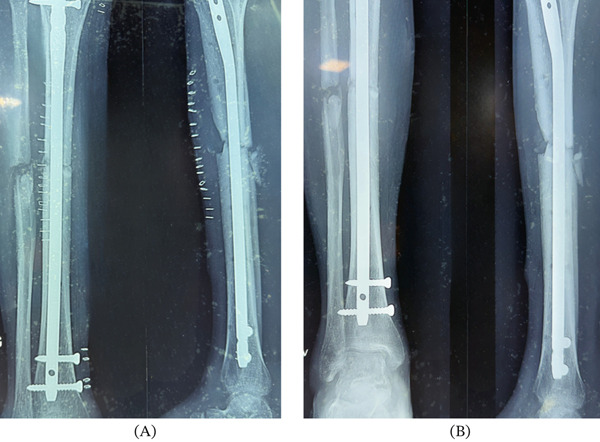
Definitive fixation with intramedullary nailing. (A) Anteroposterior and lateral radiographs dated February 17, 2025, showing intramedullary interlocking nailing with satisfactory alignment and stable fixation. (B) Anteroposterior and lateral radiographs dated March 17, 2025, showing early callus formation and progressive fracture healing.
